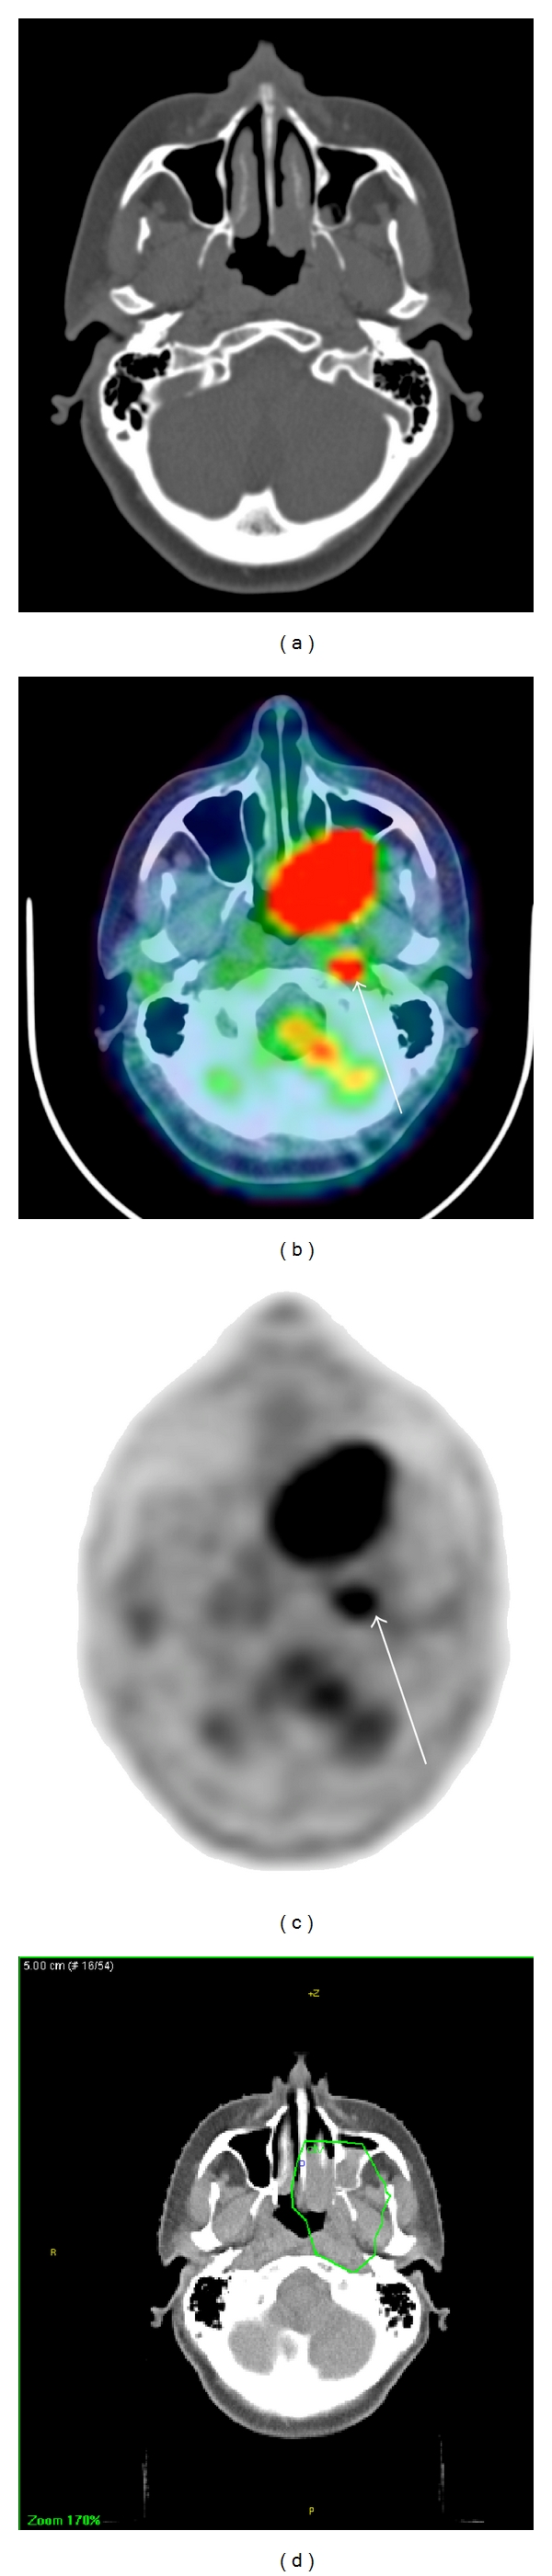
Modification of radiotherapy target volume based on FDG PET-CT. T4 maxillary sinus SCC. Diagnostic CT staged at T4 N0. FDG PET-CT identified an FDG avid (SUV_max_ = 12) left retropharyngeal lymph node altering staging to T4 N1. The radiotherapy clinical target volume incorporates this lymph node. (a) Diagnostic CT. (b) Coregistered FDG PET-CT. (c) FDG PET. (d) Green line = clinical target volume on planning CT scan including FDG avid left retropharyngeal lymph node. White arrow = FDG avid left retropharyngeal lymph node.
